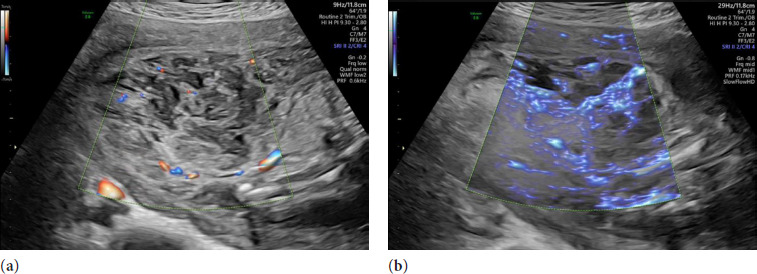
Ultrasound findings of placenta: (a) Placental lacunae consisting of an irregular area of low echogenicity in the placental parenchyma; (b) *Superb microvascular imaging* (SMI) of the overperfused placental area
